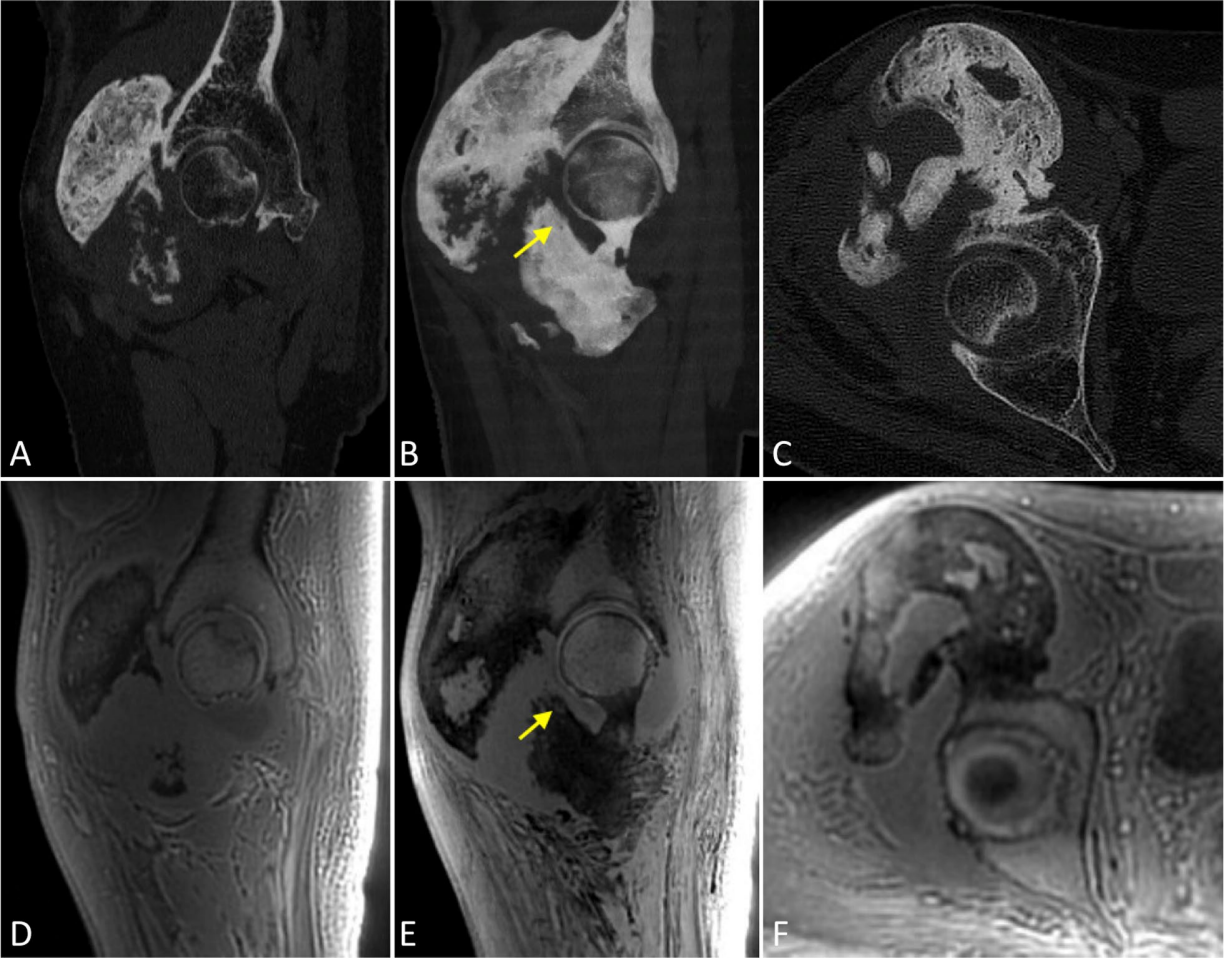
Voluminous anterior NHO oh the right hip with contact between the heterotopic ossifications and the joint capsule (arrow). Sagittal (A, B) and axial (C) CT images and their corresponding images on MRI in ZTE sequence (D–F)
